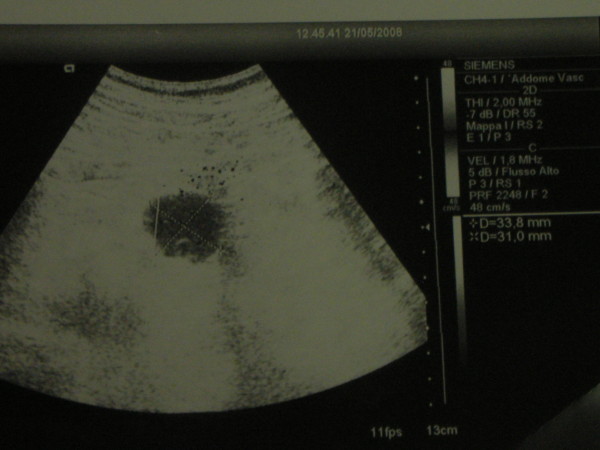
Doppler sonography showing a thrombosis of a splenic artery aneurysm.
